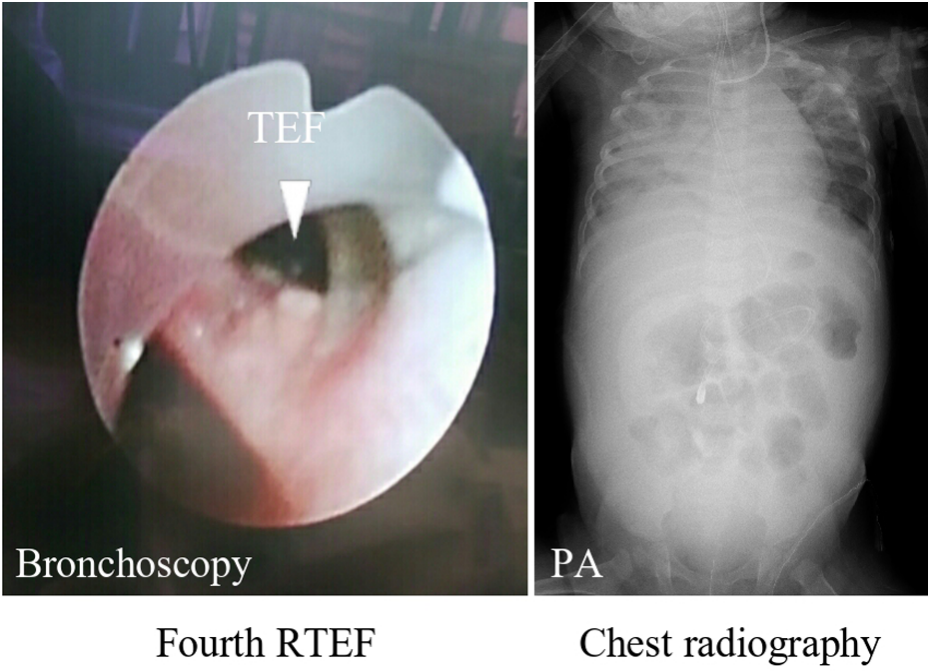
Bronchoscopy shows the fourth RTEF with a big hole (arrowhead). Chest radiography shows severe mediastinitis and right pneumonia. RTEF, recurrent tracheoesophageal fistula.
